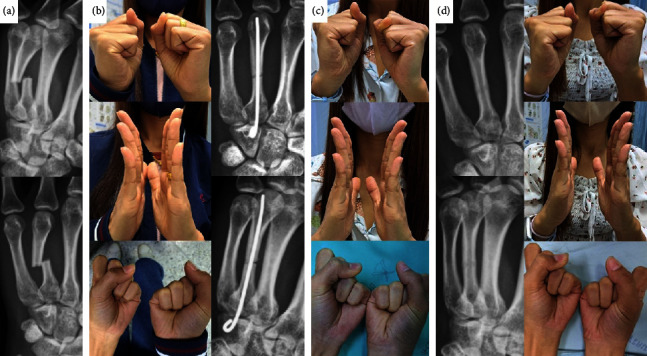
A 23-year-old female with fracture of the fourth metacarpal shaft of her left hand: (a) preoperative radiographs, (b) postoperative radiographs and clinical pictures of her hand motion at 2 weeks, (c) the patient regained full ROM at 6 weeks after the operation, and (d) uneventful bone healing and excellent functional outcomes were observed at 5 months. Her grip strength was 86.48% and the DASH score was zero. She denied further appointment.
